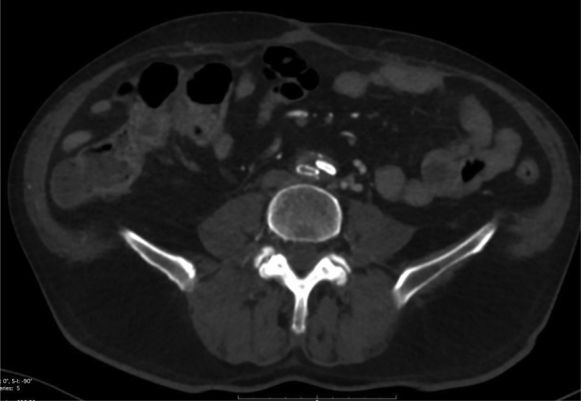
Computed tomography scan showing the right stent crushed and occluded and the left stent partially collapsed but patent.
